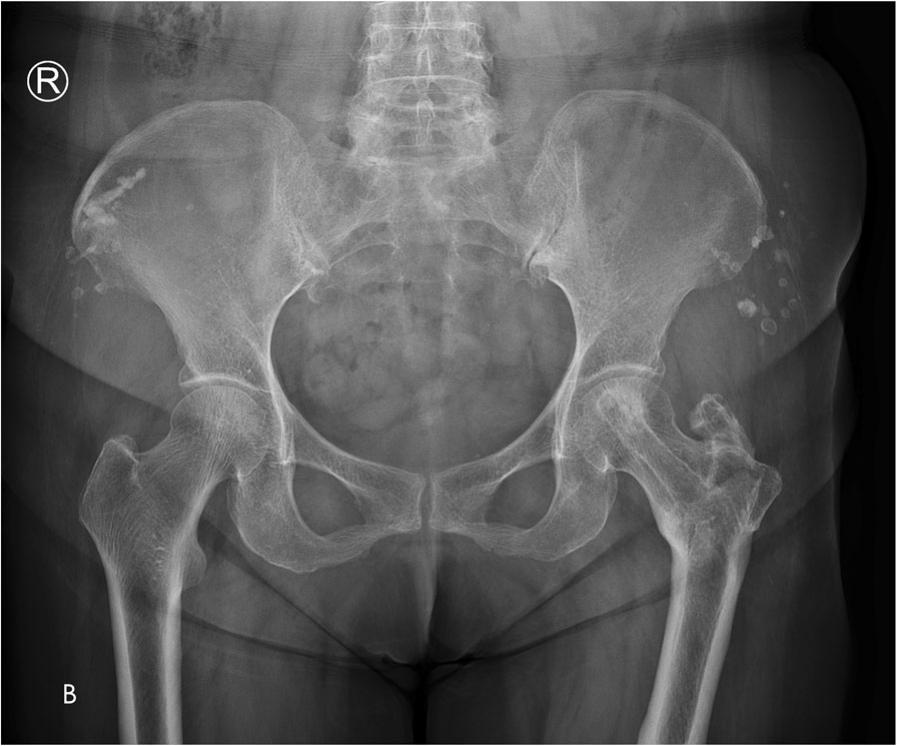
The anteroposterior radiograph of the pelvis after the PFNA removal
